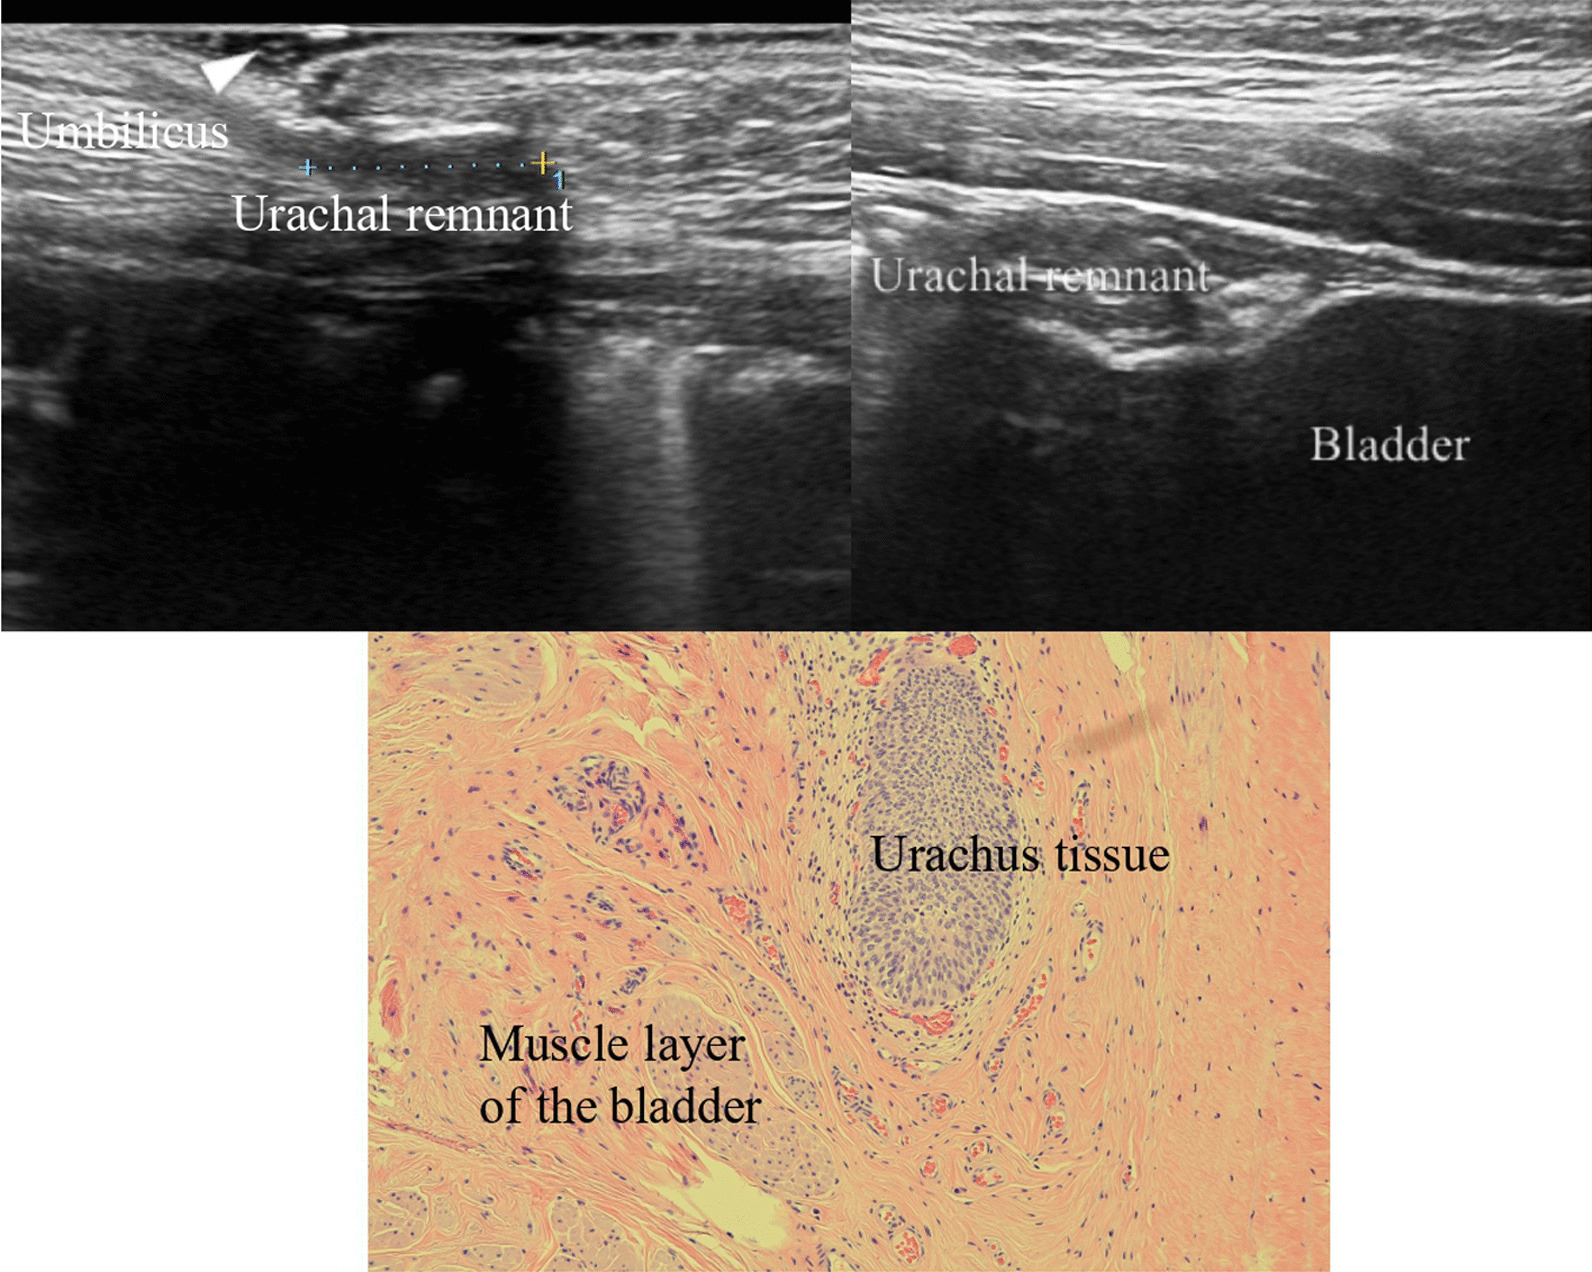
Ultrasonography shows that the urachal remnant continued toward the bladder apex. Pathological findings indicate urachus tissue at the bladder apex
